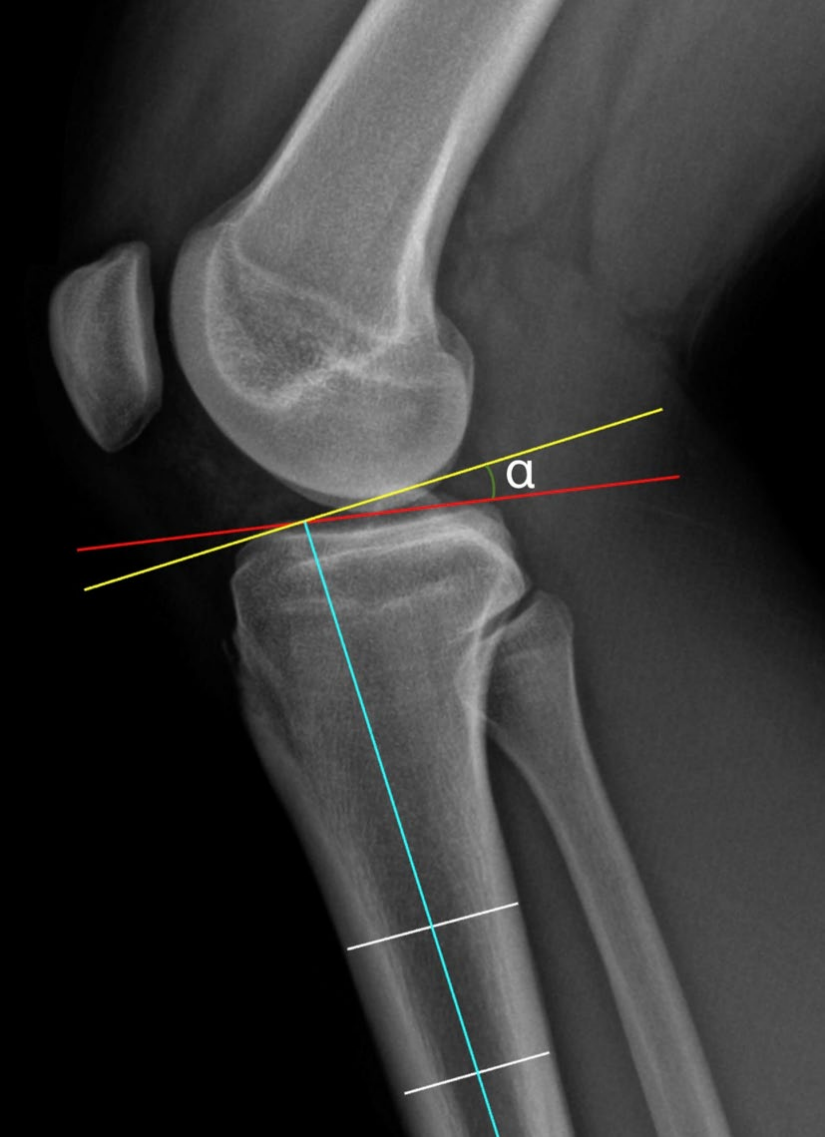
True lateral’ radiograph of a knee. The posterior tibial slope (PTS) is the angle (*α*) between the perpendicular (yellow line) to the tibial longitudinal axis (light blue line) and the tangent to the anterior and posterior edges of the medial tibial plateau (red line) as described by Dejour et al. [11] (colour figure online)
